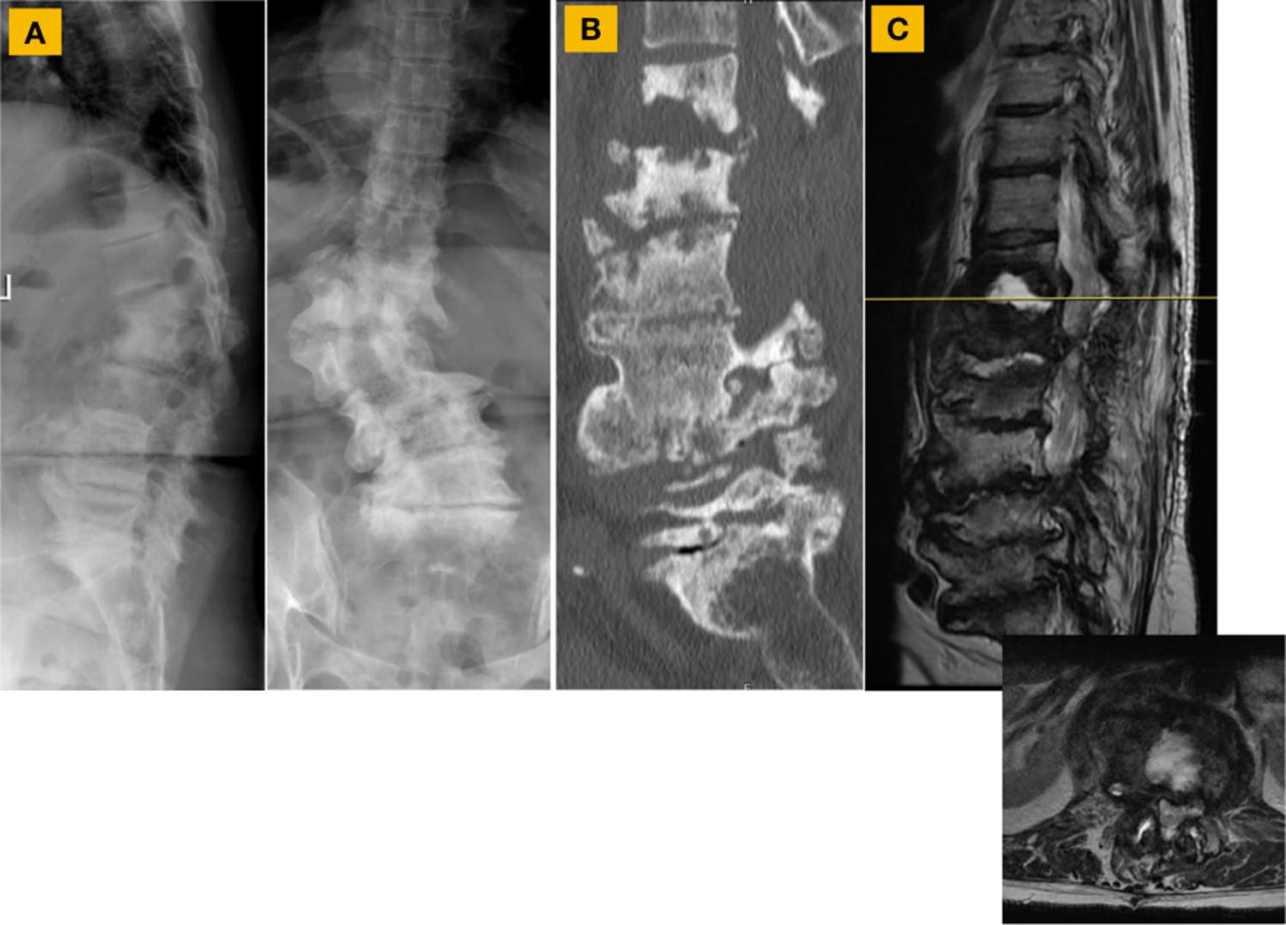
A Presenting radiographs demonstrated degenerative scoliosis, flatback, and pathological vertebral body fractures with posterior element subluxation at T12–L1 resulting in thoracolumbar kyphosis. B Computed Tomography (CT) confirmed the T12 and L1 pathological fractures but also showed flexibility of the segmental kyphosis. C Magnetic resonance imaging of the lumbar spine revealed severe central stenosis at T12–L1, severe lateral recess and foraminal stenosis from T12–S1 bilaterally, and a prior narrow T12–L4 decompression
